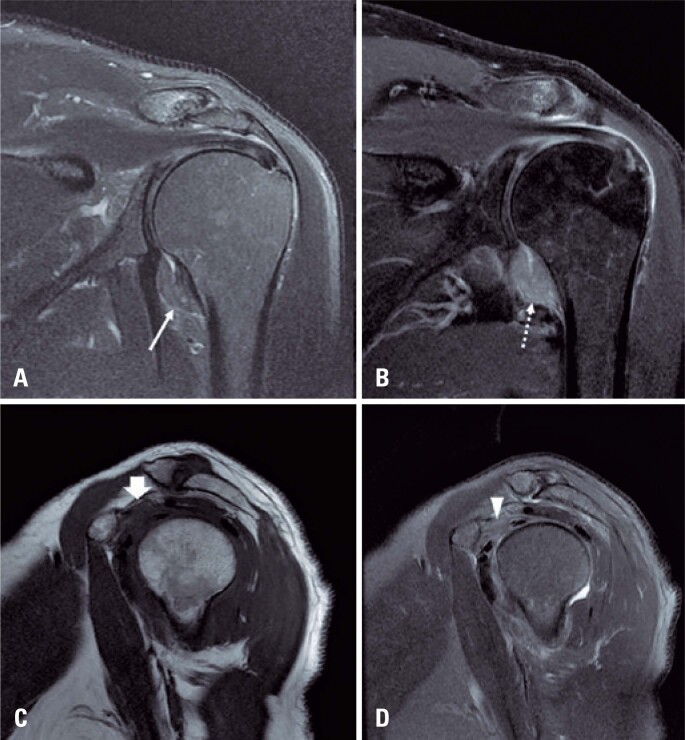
Typical case of adhesive capsulitis in a 64-year-old male patient with shoulder pain for one month, without trauma. Coronal T2 fat suppression-weighted-image (A) shows glenohumeral capsular thickening and pericapsular edema affecting the axillary recess (arrow). Coronal T1-weighted-image post gadolinium with fat suppression (B) shows glenohumeral capsular thickening and capsular and pericapsular enhancement affecting the axillary recess (dashed arrow). Sagittal T1-weighted-image (C) and T2-weighted-image with fat suppression (D) shows obliteration of the pericapsular fat tissue along the rotator interval region (short arrow and arrowhead)
